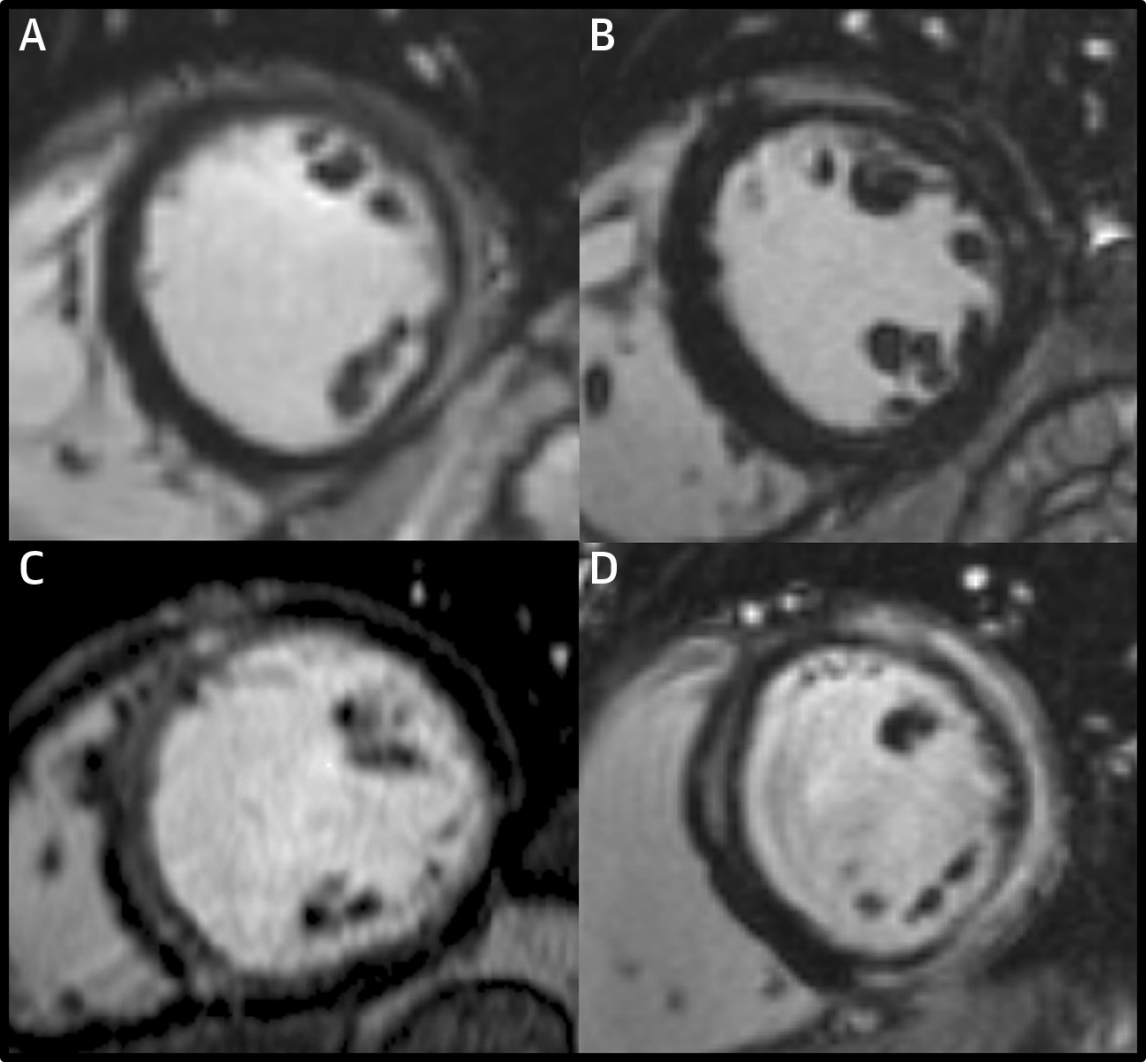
Typical MRI Late Gadolinium Enhancement Images Mid-ventricular short-axis views for 4 patients with normal indexed left ventricular volumes, wall thickness and ejection fraction demonstrating; (A) sub-epicardial enhancement in the inferolateral wall, (B) sub-epicardial enhancement in the anterolateral wall, (C) linear mid-wall enhancement in the septum, (D) mid-wall enhancement of the septum and sub-epicardial enhancement of the anterior, lateral and inferior walls. MRI = magnetic resonance.
